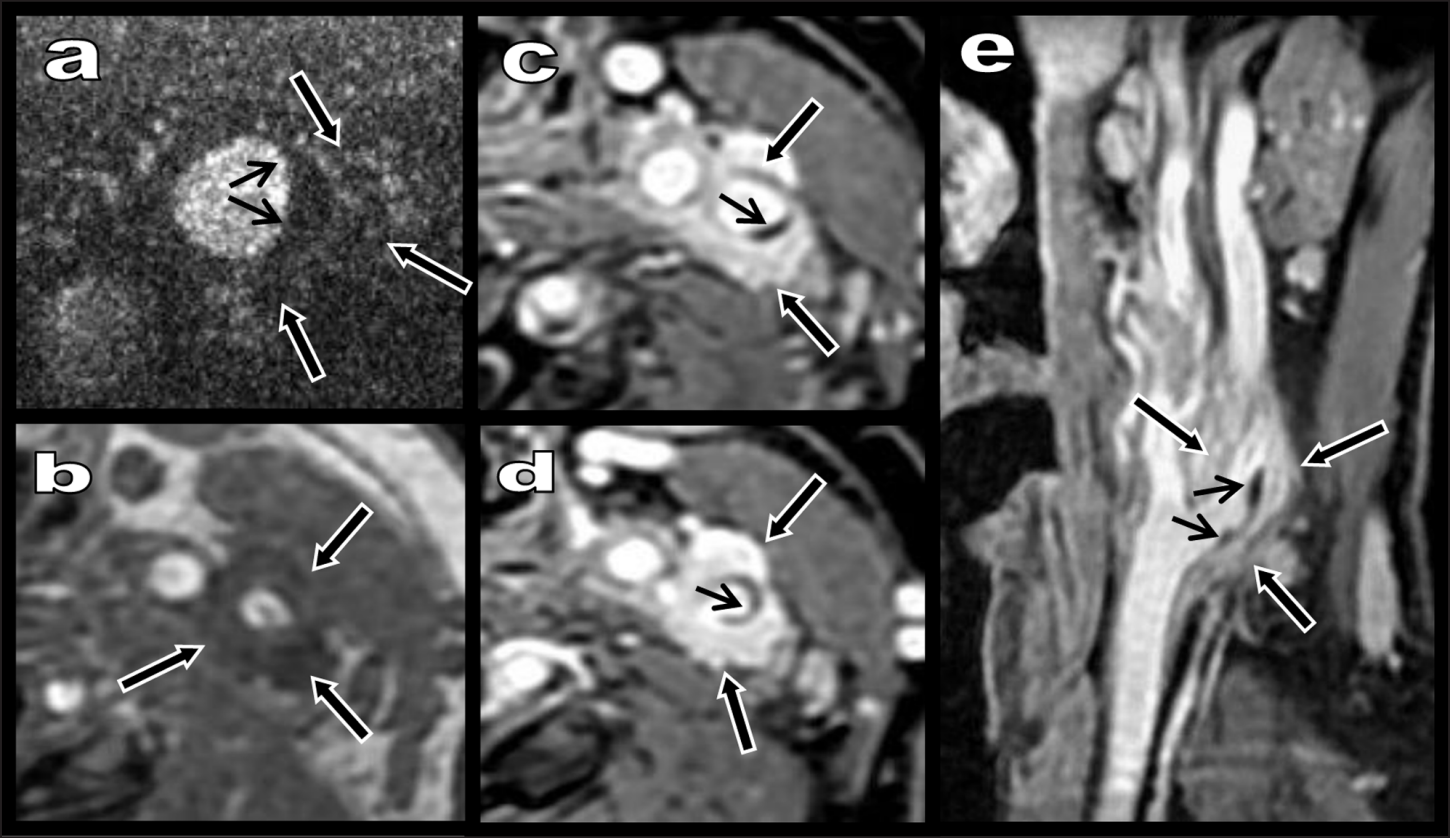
Contrast-enhanced ultrasound (a) show normal capillary distribution of micro bubbles in the hypoechoic thickening (black arrows) excluding haematoma. The avascular intimal fibrous plaque is clearly seen (small black arrows). Unenhanced Axial T1-weighted MR image (2) shows a hypointense tissue (black arrows on a–e) around the proximal internal carotid. Intense enhancement of this tissue appears on fat-saturated contrast enhanced T1-weighted images (c–e).
